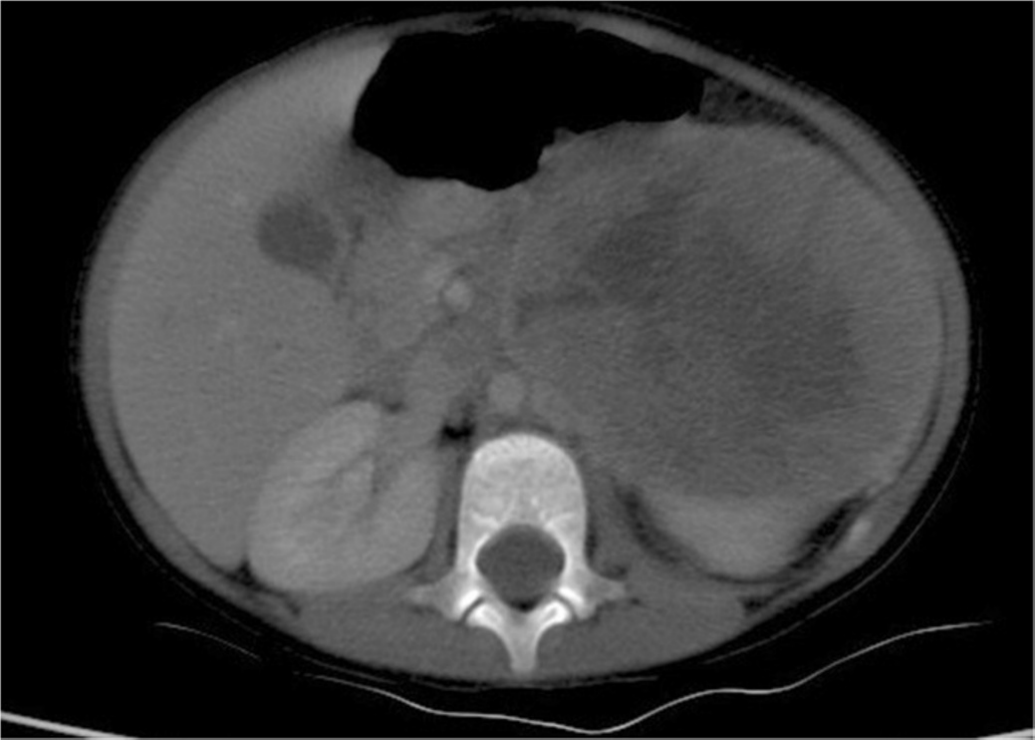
Patient 1: Magnetic resonance imaging shows a large heterogeneous mass arising from the left adrenal gland associated with heterogeneous enhancement of the tumor thrombus in the inferior vena cava (arrow).
